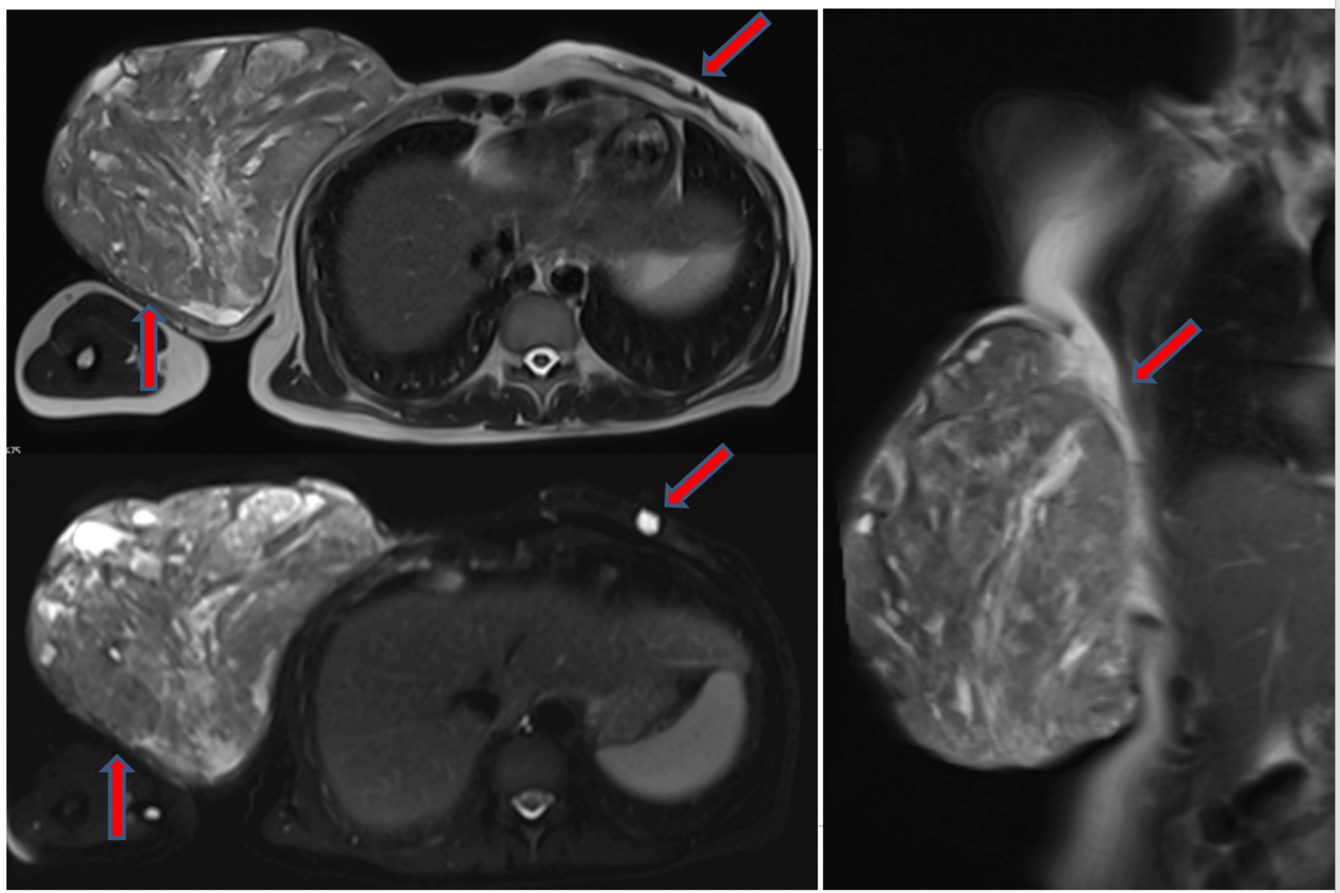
Right and left MRI images of bilateral phyllodes tumor
